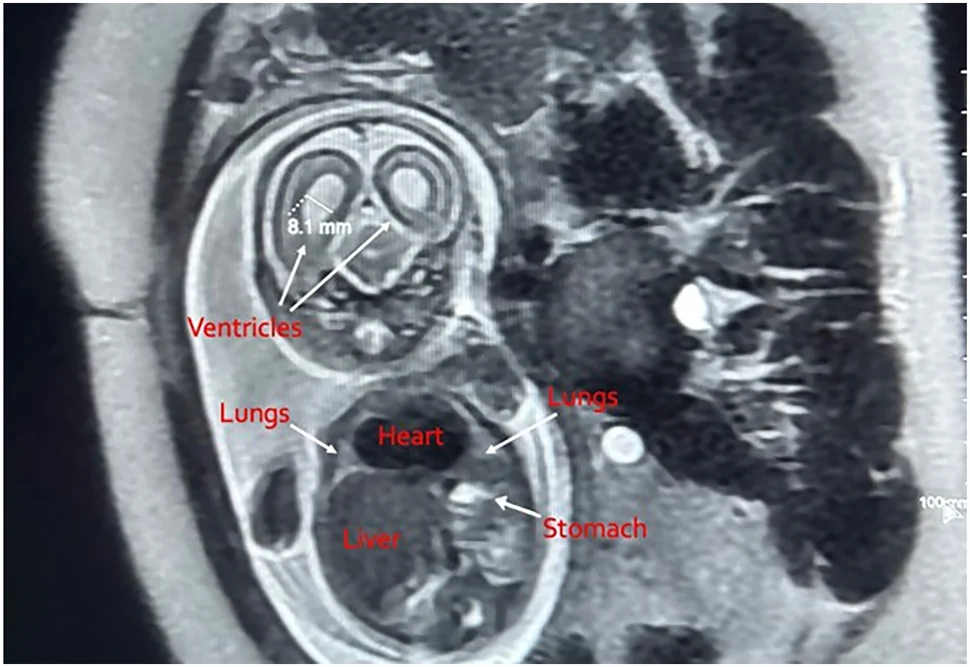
Fetal MRI showing cerebral ventriculomegaly, small stomach, normal amniotic fluid volume.
